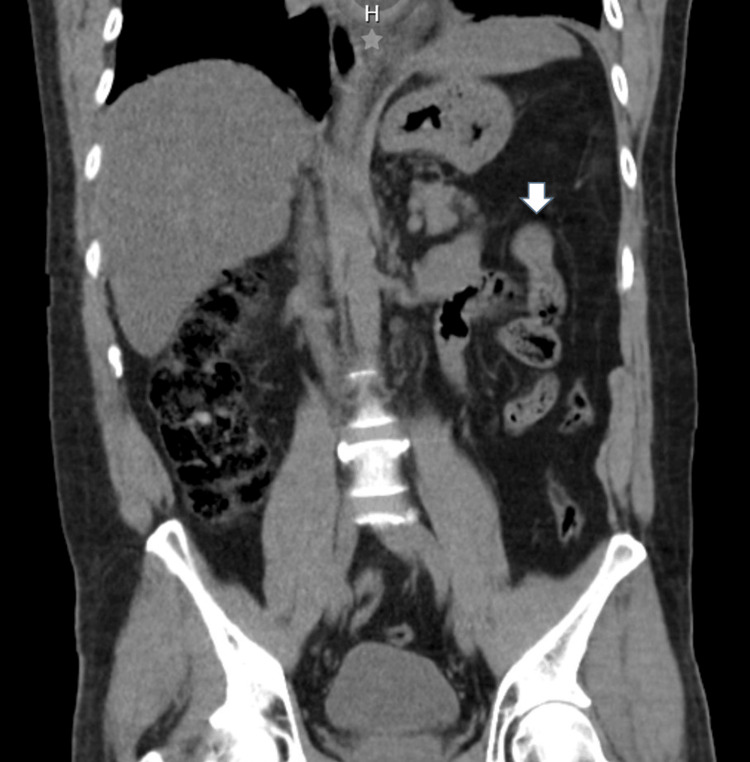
CT scan revealing heterogeneous tubular image in the small bowel (white arrow)
